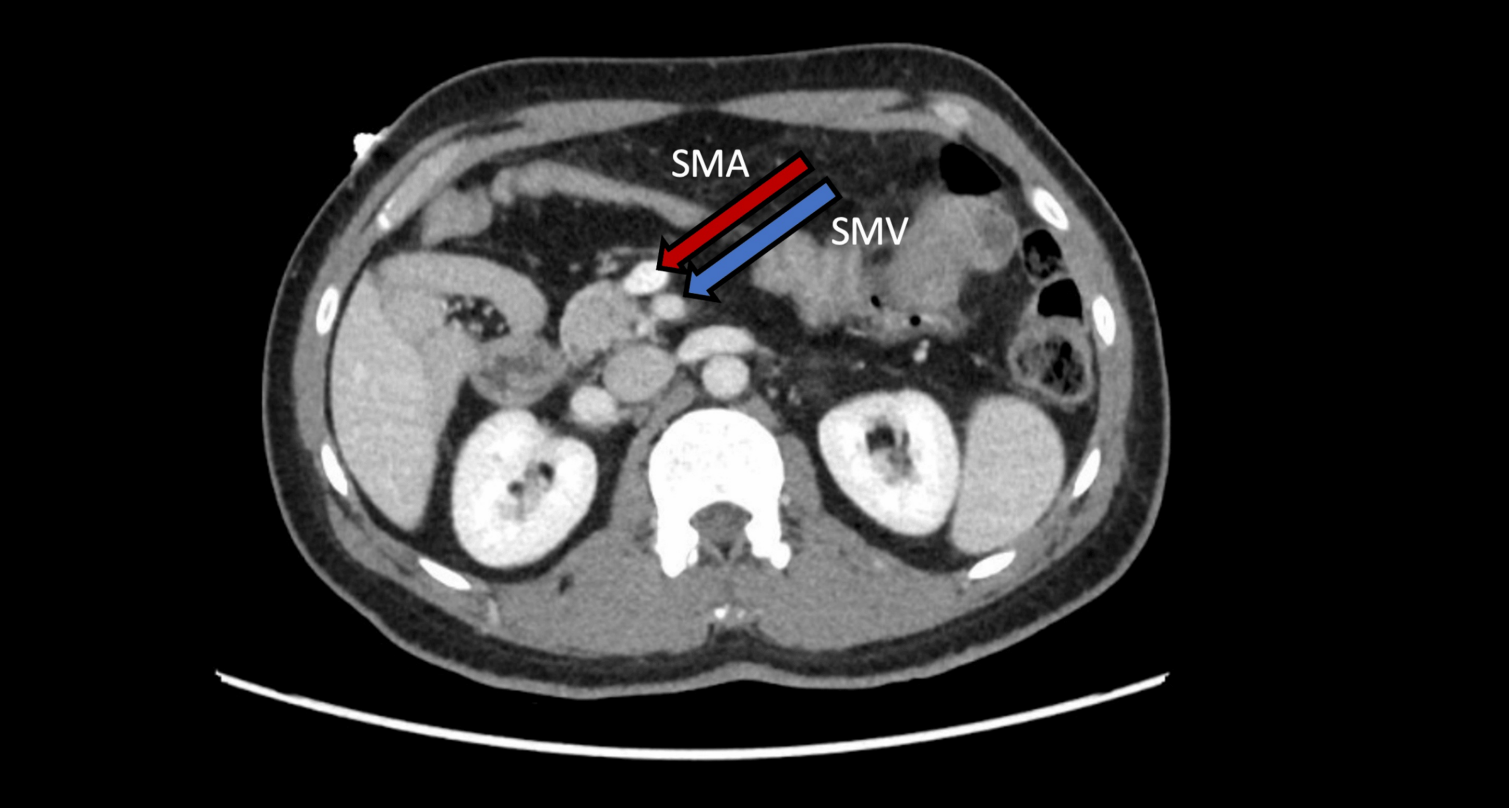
Vertical orientation of the superior mesenteric vessels Axial CT image demonstrating the superior mesenteric artery (SMA) and superior mesenteric vein (SMV) that exhibit a vertical configuration. Under normal anatomy, the SMA lies to the left of the SMV in a horizontal configuration. A vertical SMA-SMV orientation is suggestive of intestinal malrotation. CT, computed tomography.
